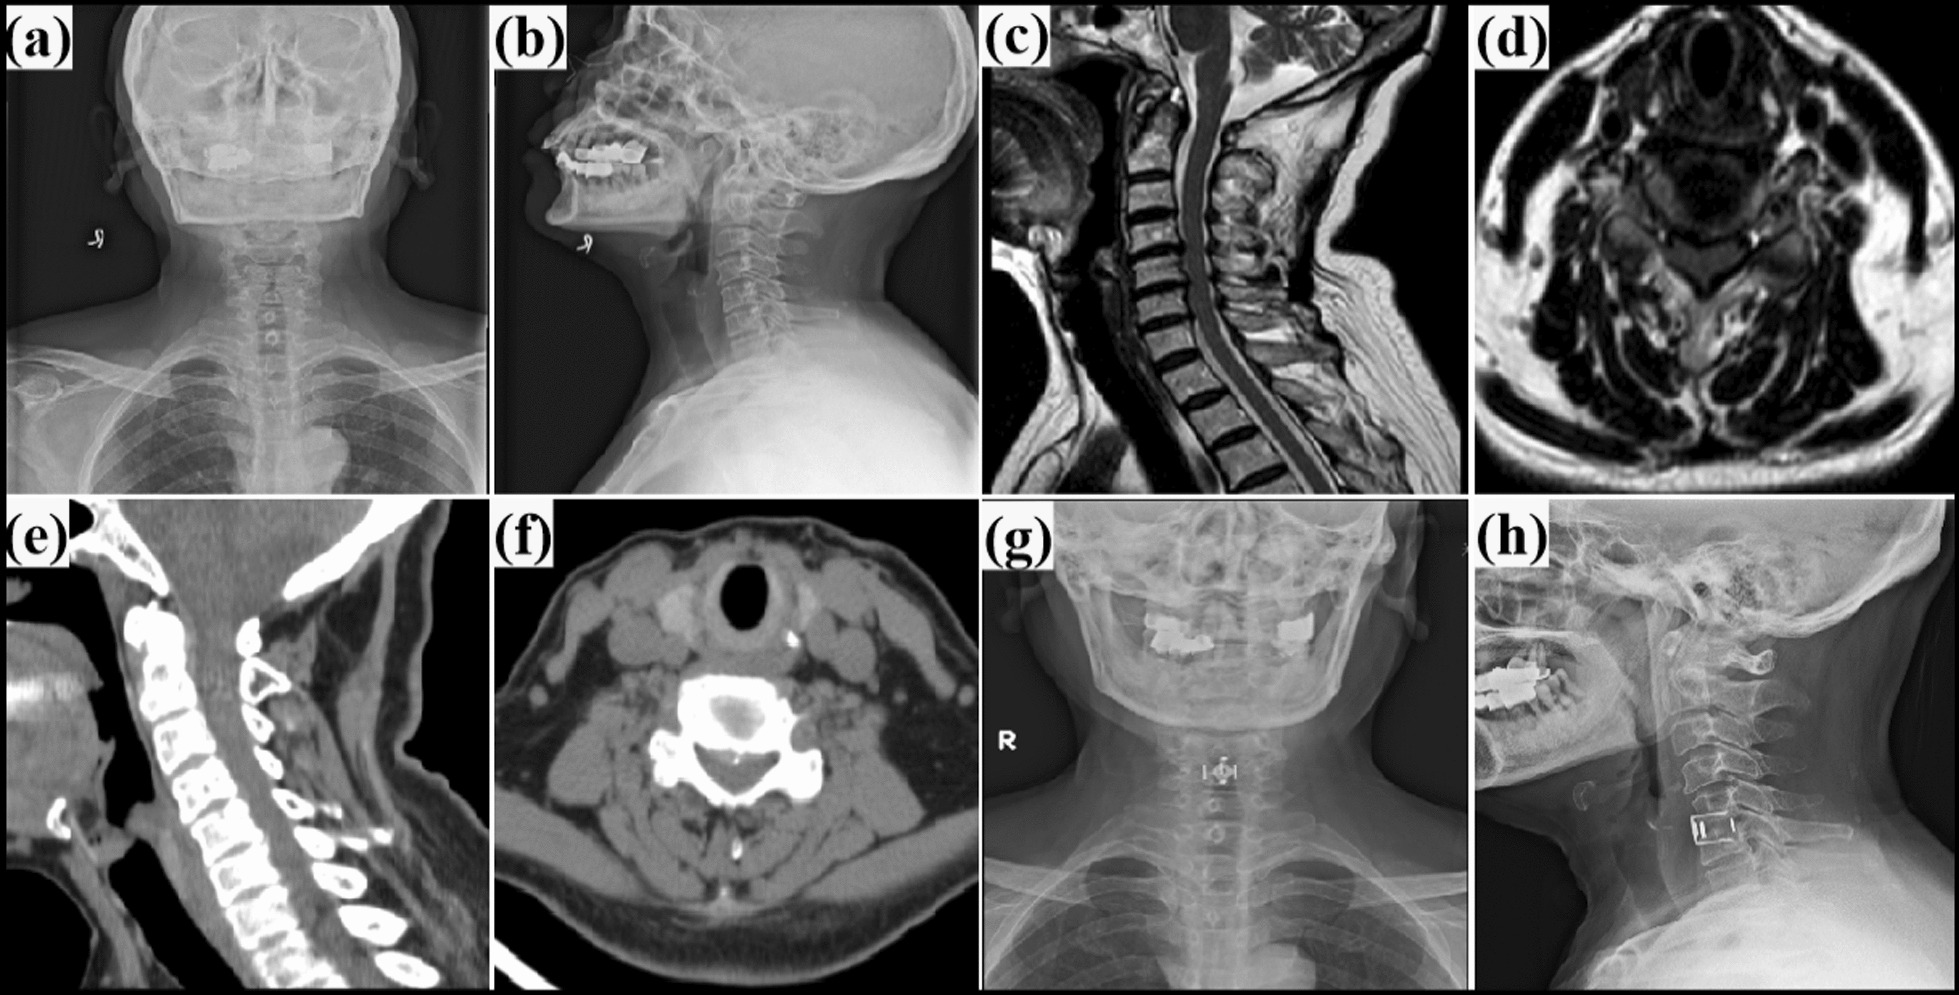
A Clinical Application Example of the Door-Shaped Titanium Plate: a, b Preoperative cervical X-ray images in anteroposterior and lateral views; c, d Preoperative cervical MRI images, e–f preoperative cervical CT images, and g, h Postoperative cervical X-ray images taken one week after the procedure
